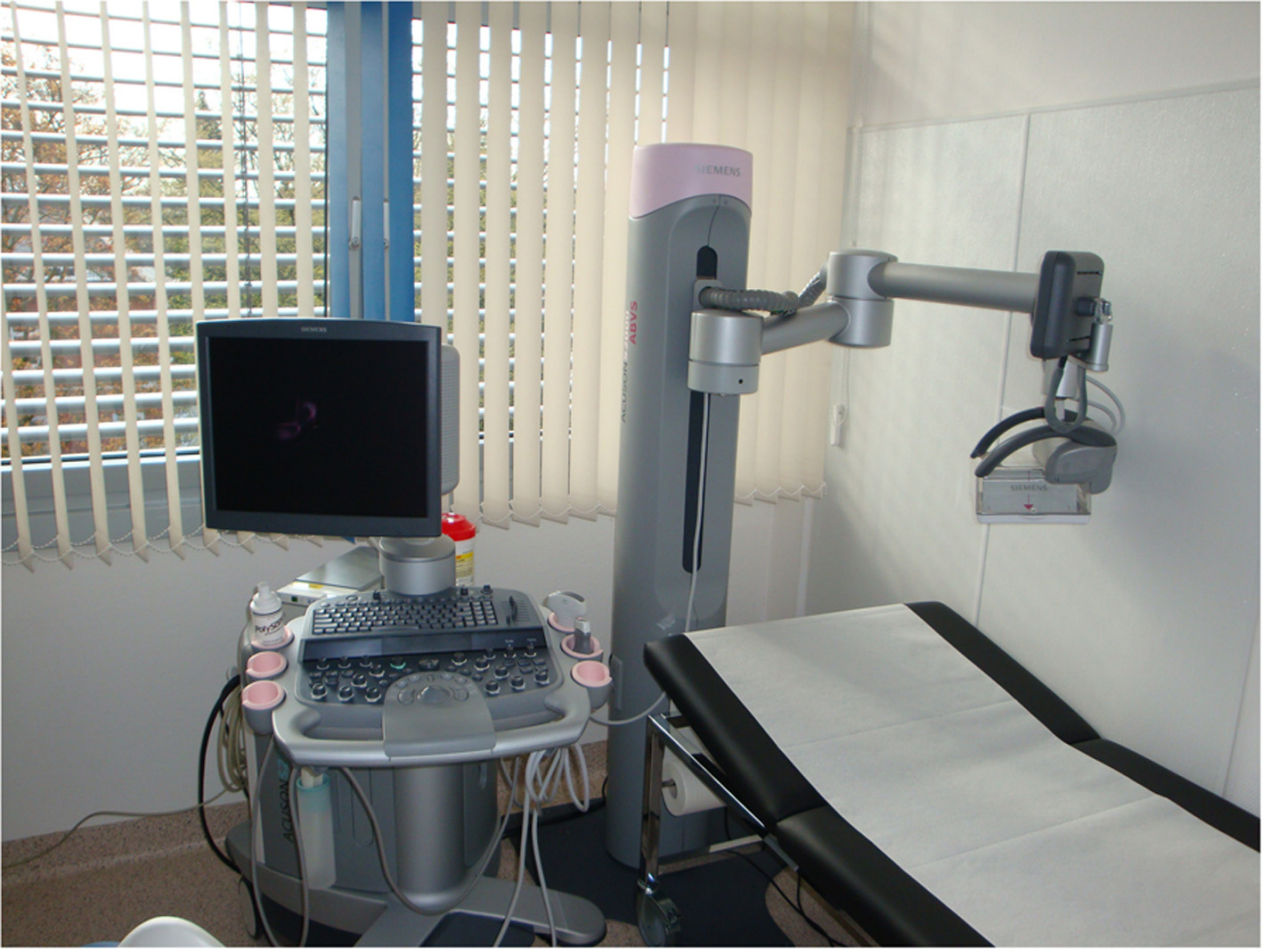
ACUSON S2000™ ABVS. On the left-hand side is the ACUSON S2000™ ultrasound machine, on the right-hand side is the 14L5BV volume transducer attached to a mechanical arm.
